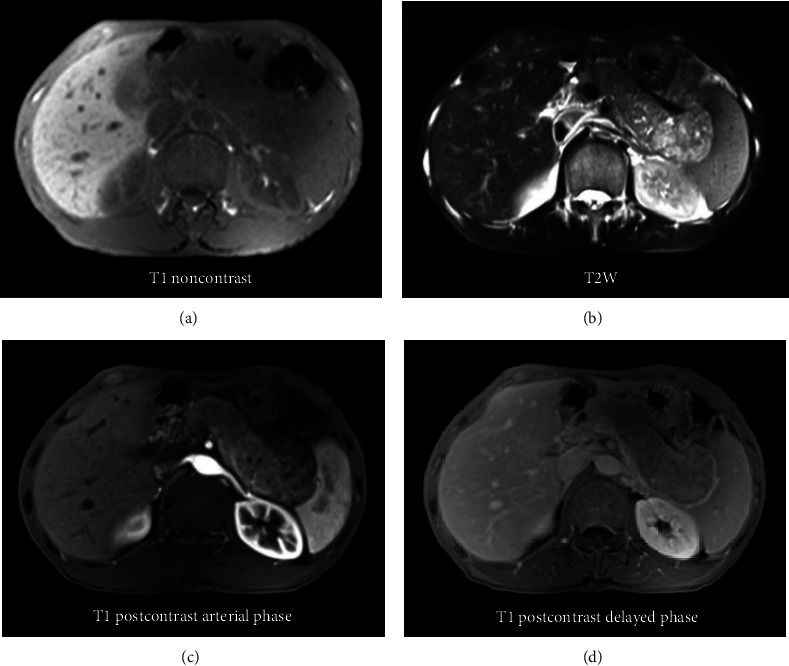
MRI on T1-weighted image (a), T2-weighted image (b), and arterial phase (c) and delayed phase (d) in T1-weighted image with fat-sat after contrast administration. MRI shows a diffusely enlarged pancreas with low intensity on T1-weighted imaging and heterogeneous high intensity on T2-weighted imaging, particularly in the body and tail region of the pancreas. The pancreas also shows remarkable enhancement in the arterial phase and washout in the delayed phase.
